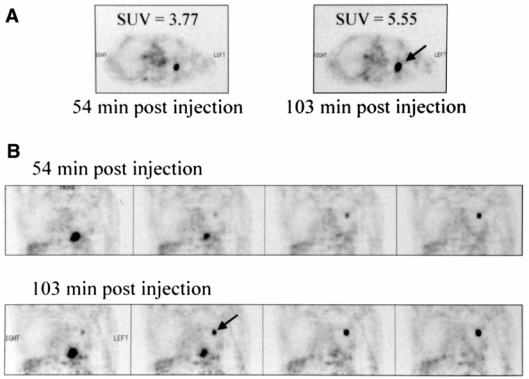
Comparison of early and delayed FDG-PET images from a lung cancer patient. transaxial images (A) and coronal images (B). Arrow points to lesion. Malignant focus became more apparent in later images and SUV increased from 3.77 to 5.55. Reprinted with permission from [56].
